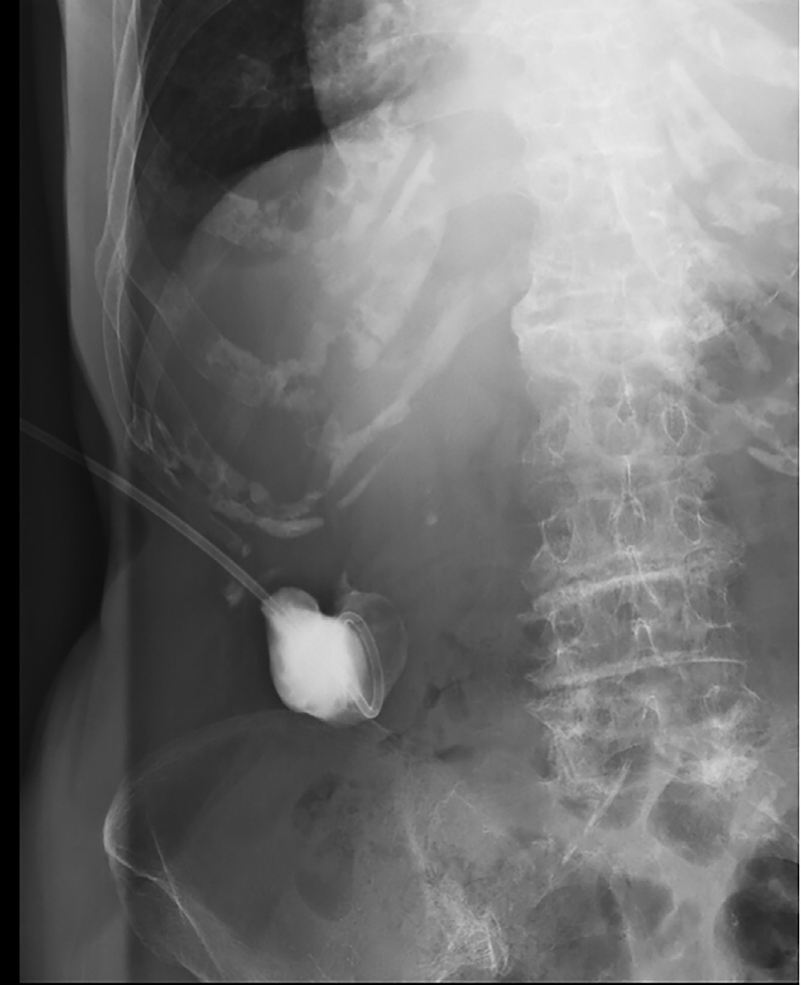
Contrast image confirming the drainage abscess cavity. An abdominal X-ray shows the drainage abscess cavity (Fig. 2).
